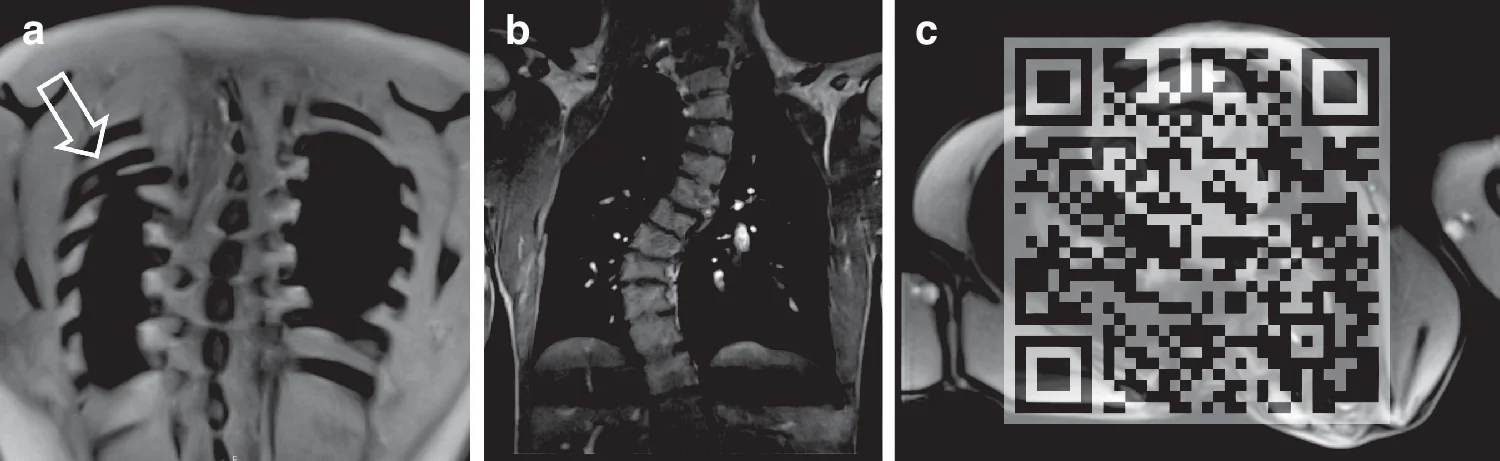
Anatomical deformities after open EA repair. a Fusion of Rib 4 and 5 (arrow) and b scoliosis in a 29- and 27-year-old patient, respectively, following open EA repair. c Real-Time MRI of a patient with severe vertebral and chest wall anomaly in 20-year-old EA patient. (https://pedz.de/supplementals/ea-adult/video1.mp4).
